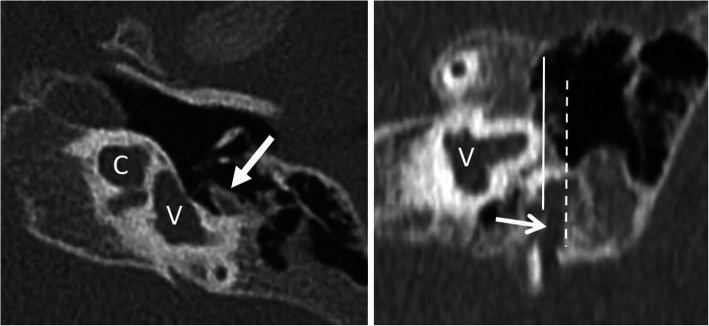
A 1-year-old male patient, with bilateral severe SNHL from birth. HRCT axial image (left image) shows incomplete partition type I with cystic cochlea (C) and vestibulum (V). The horizontal segment of the facial nerve (arrow) is lateralized. HRCT coronal image (right image) shows an interrupted line corresponding to the most lateral aspect of the vertical segment of the facial nerve (open arrow) lateral to the continuous line corresponding to the most lateral bony aspect of the lateral semicircular canal. The normal location of the vertical segment is medial to the lateral semicircular canal
